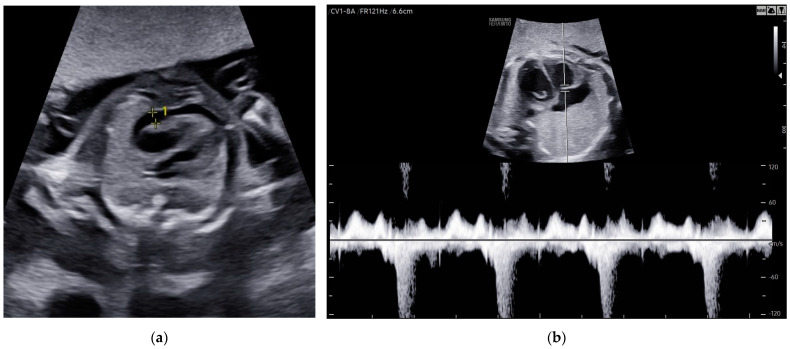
Case 7: Abnormalities in the fetal heart ultrasound: (a) pericardial effusion before the first intrauterine transfusion, 21 weeks of gestation; (b) regurgitation of the mitral valve and total resolution of pericardial effusion, 2 weeks after the second intrauterine transfusion.
